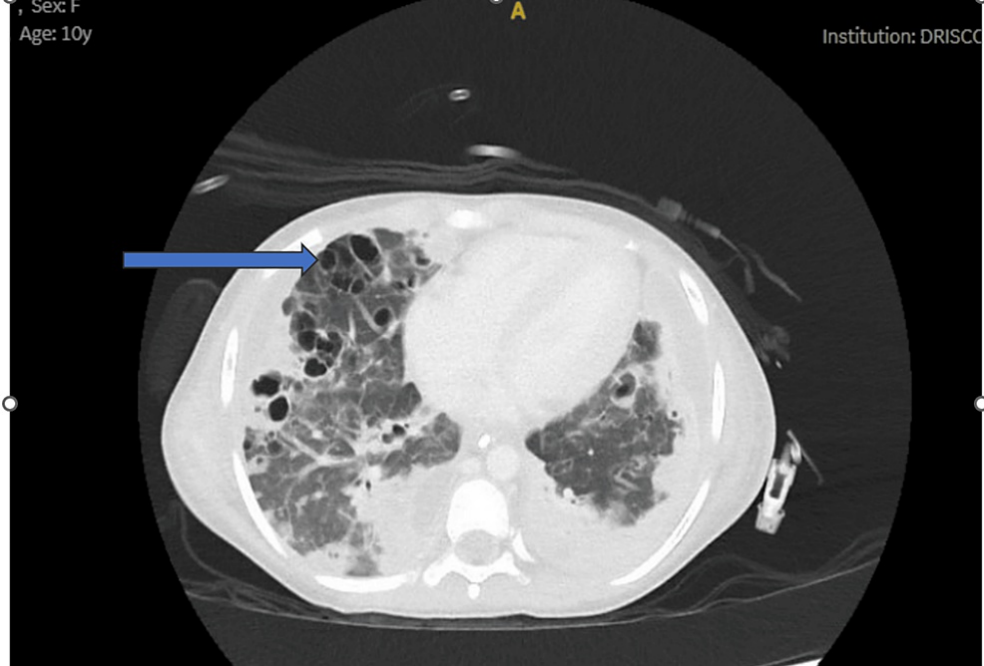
Computed tomography of the chest on admission The blue arrow shows cavitary changes in the right lung.
